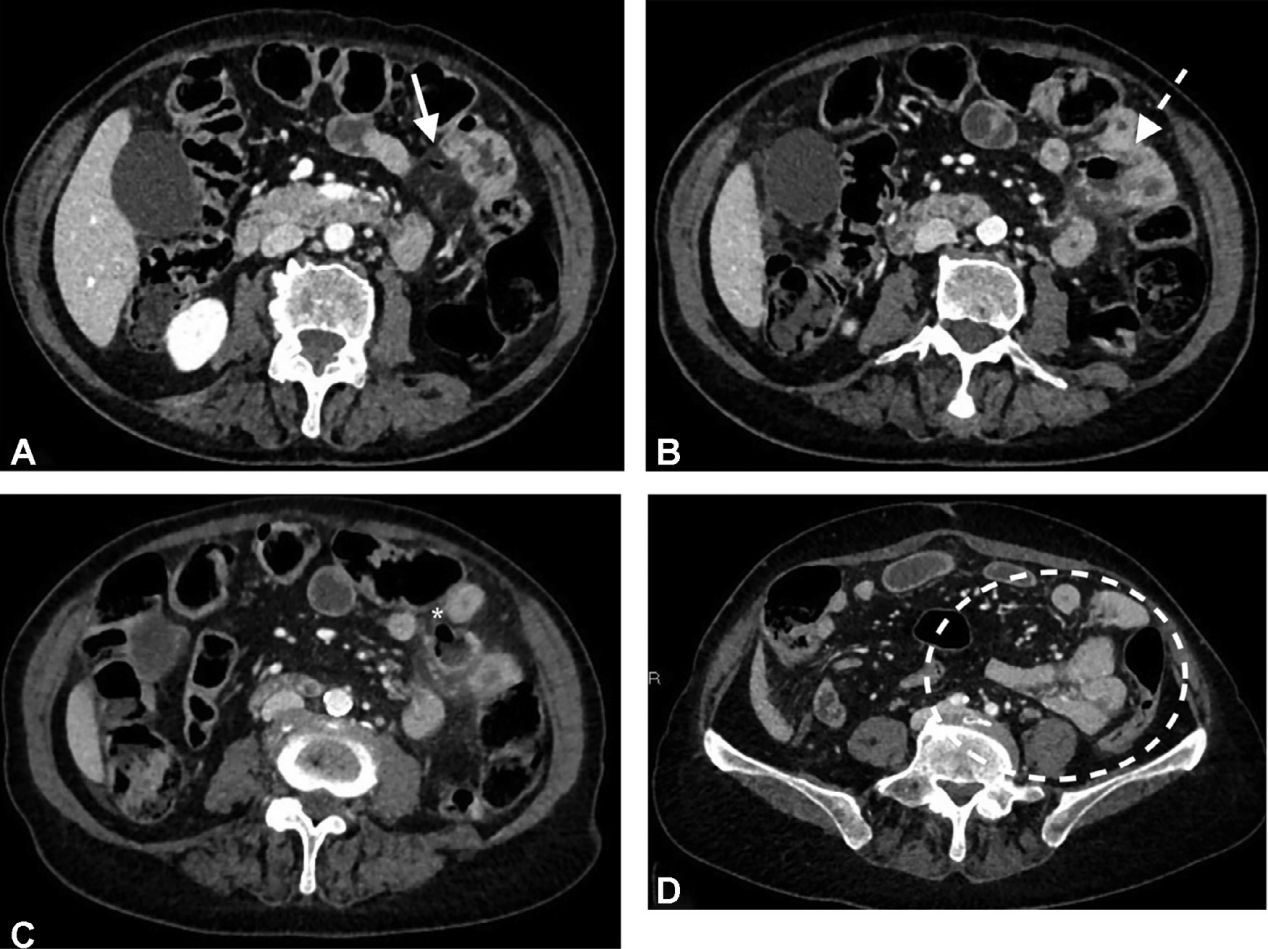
(A-D) Axial CT contrast images (parenchymal phase). A millimetric extraluminal air bubble is detected strictly close to the mesenteric side of the jejunal loop (white arrow) (A) The out-pouching lesion is detected in connection with the jejunum. The diverticular sac is air filled with thick walls (dashed arrow) (B) On the anterior side of the diverticular sac the wall is not appreciable (asterix) (C) The adjacent bowel presents eccentric thickened and hyperemic walls. Other jejunal loops are collapsed and angled resembling identically the US findings (dashed circle) (D) Stranding of peri-diverticular sac is visible on the mesenteric side.
